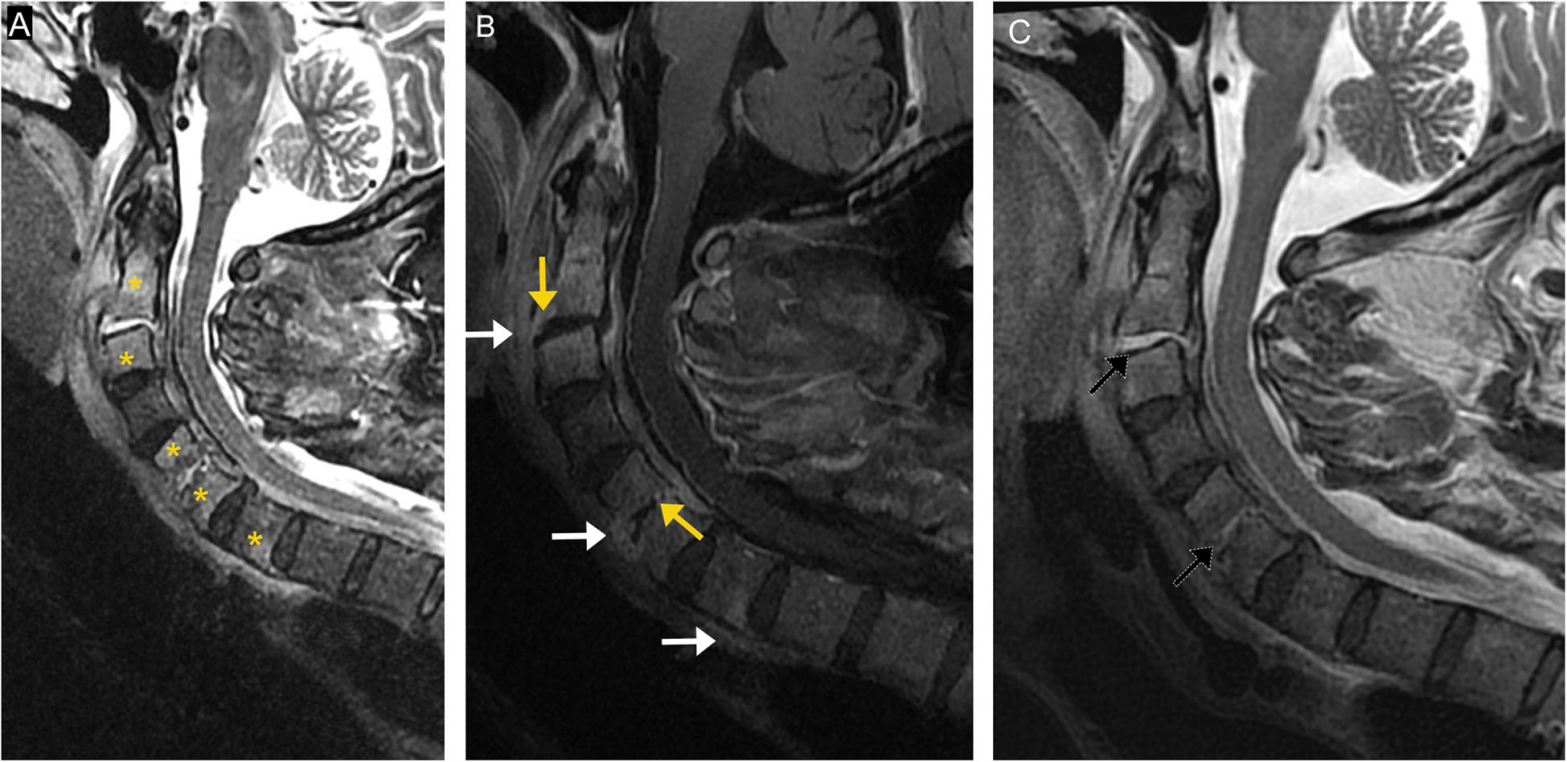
Pyogenic spondylodiskitis. Sagittal STIR image (a) demonstrates abnormal marrow signal of C2, C3, C5, C6, and C7 (asterisks) with diffuse paravertebral soft tissue edema. Post-contrast T1-weighted image (b) demonstrates corresponding marrow enhancement, disc enhancement, and endplate erosion (orange arrows). Phlegmon or abscesses are seen at the level of C2-C3, C5-C6, and C6-C7 (white arrows), as well as epidural phlegmon extending along the dorsal aspects of C2 to C7. Sagittal T2 image (c) demonstrates fluid signal within the disc at C2-C3 and C5-C6 (black arrows), characteristic of pyogenic spondylodiskitis
